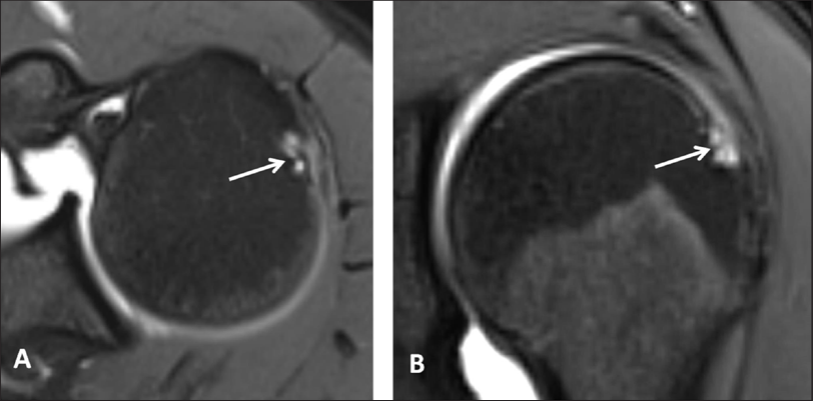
Subchondral cysts of the humeral head and normal bare area. (A) Axial and (B) Coronal oblique fat-suppressed T1-weighted MR arthrographic images show subchondral cysts at the attachment of the infraspinatus tendon (arrow). Coronal oblique section of the same patient discloses a normal bare area in the posterolateral aspect of the humeral head with small fibrocystic changes that communicate with the joint and should not be mistaken for a cartilage defect (arrow, B). Such changes are common and often asymptomatic.
